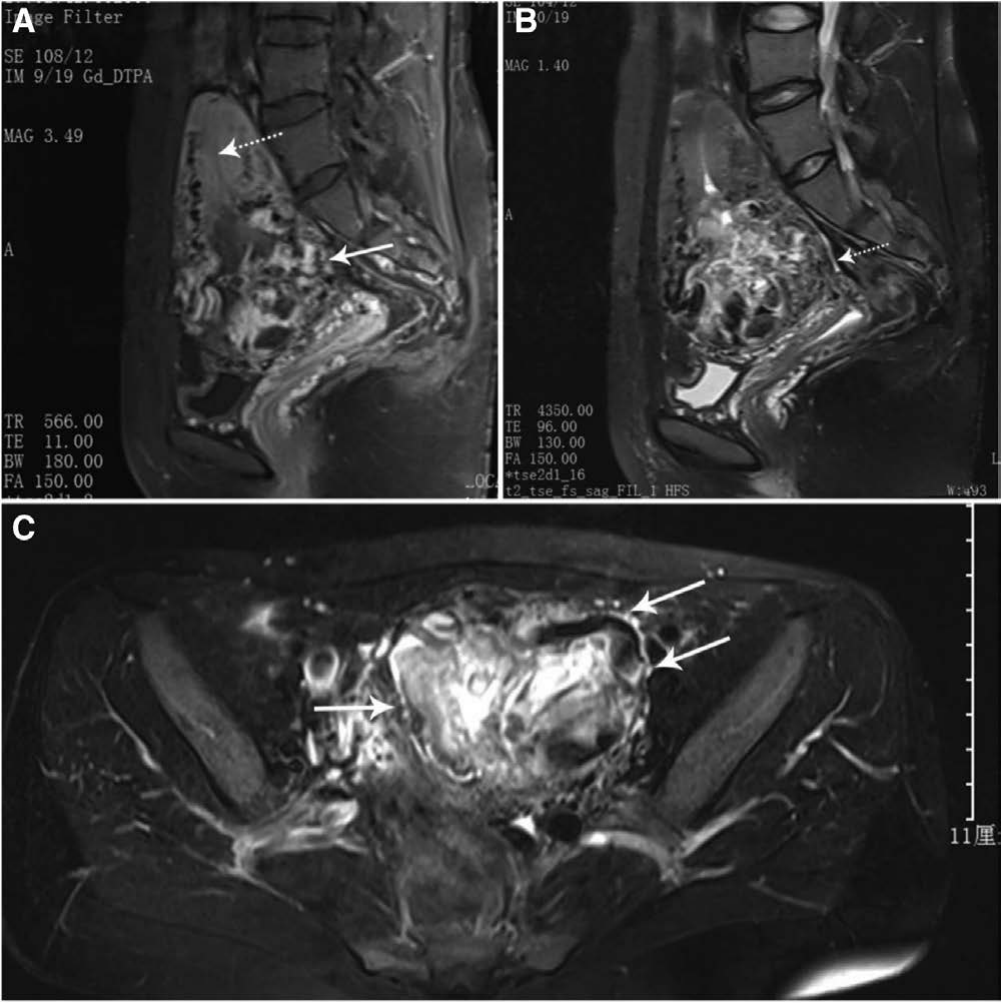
MRI images for the CSP patient. (A) Sagittal T1-weighted MRI showing a uterine mass (solid arrow) at the C-section scar with an empty uterine cavity (dashed arrow). (B) Sagittal T2-weighted MRI showing that the mass has some complex echo spaces that were due to hemorrhage or necrotic tissue, cysts, or vascular spaces (dashed arrow). (C) Coronal T2-weighted MRI showing the mass with abundant blood flow signals (solid arrow). CSP = cesarean section scar pregnancy, MRI = magnetic resonance imaging.
